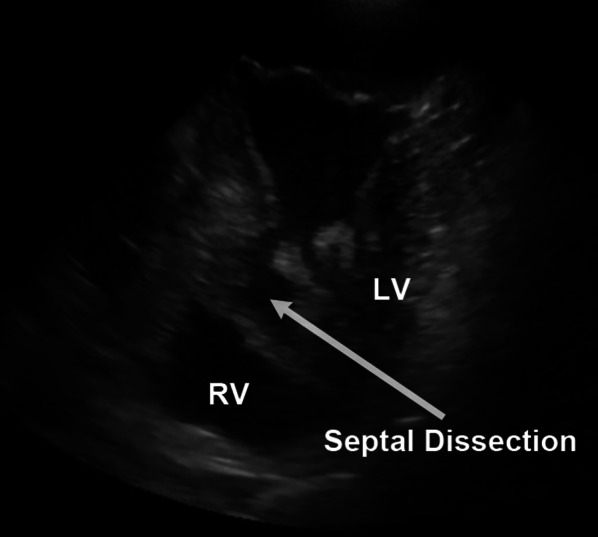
Transesophageal echocardiogram. A ventricular septal dissection is shown with communication to the left ventricle via a small orifice-generating aneurysm. *LV* left ventricle, *RV* right ventricle
